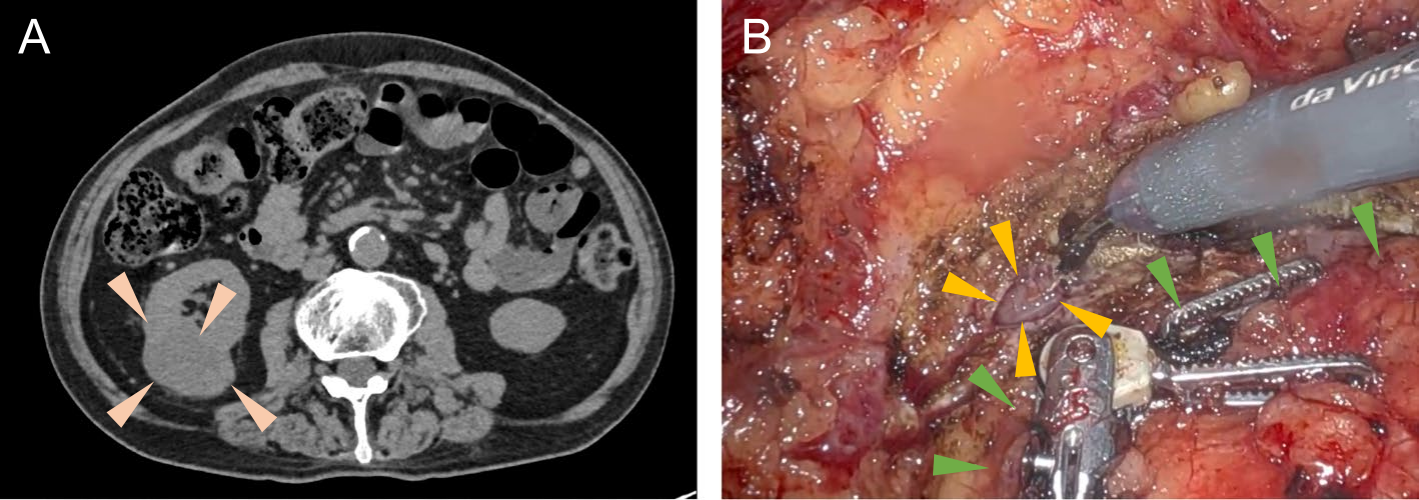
Preoperative and intraoperative images of the renal tumor. A Preoperative abdominal CT scan showed a tumor located at the lower pole of the right kidney. B Intraoperative photograph showing the injured vein. The robotic surgery was performed using the da Vinci Xi surgical system®. The tumor (green arrowheads) was retracted with a fenestrated grasper, and its margins were dissected using monopolar curved scissors. During the procedure, although no injury to large veins, such as the inferior vena cava and renal vein, occurred, a dilated vein located at the margin of the renal tumor (yellow arrowheads) was injured during tumor resection, and remained open for 22 min
